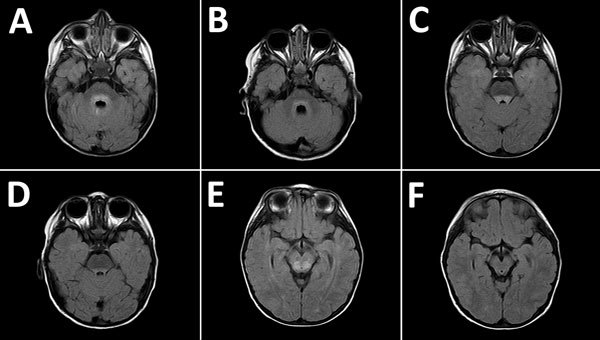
Magnetic resonance images of the brain of a 2-year-old boy with enterovirus meningoencephalitis. A–C) Brain at time of diagnosis. FLAIR sequences show hyperintense lesions around ventricle IV (A), posterior region of the pons (B), and posterior region of the mesencephalon (C). D–F) Control images of cerebrum 6 months after diagnosis. FLAIR sequences show slight hyperintensity of signal around ventricle IV, lower than in the initial study (D), and complete resolution of lesions in the posterior region of the pons (E) and mesencephalon (F).
